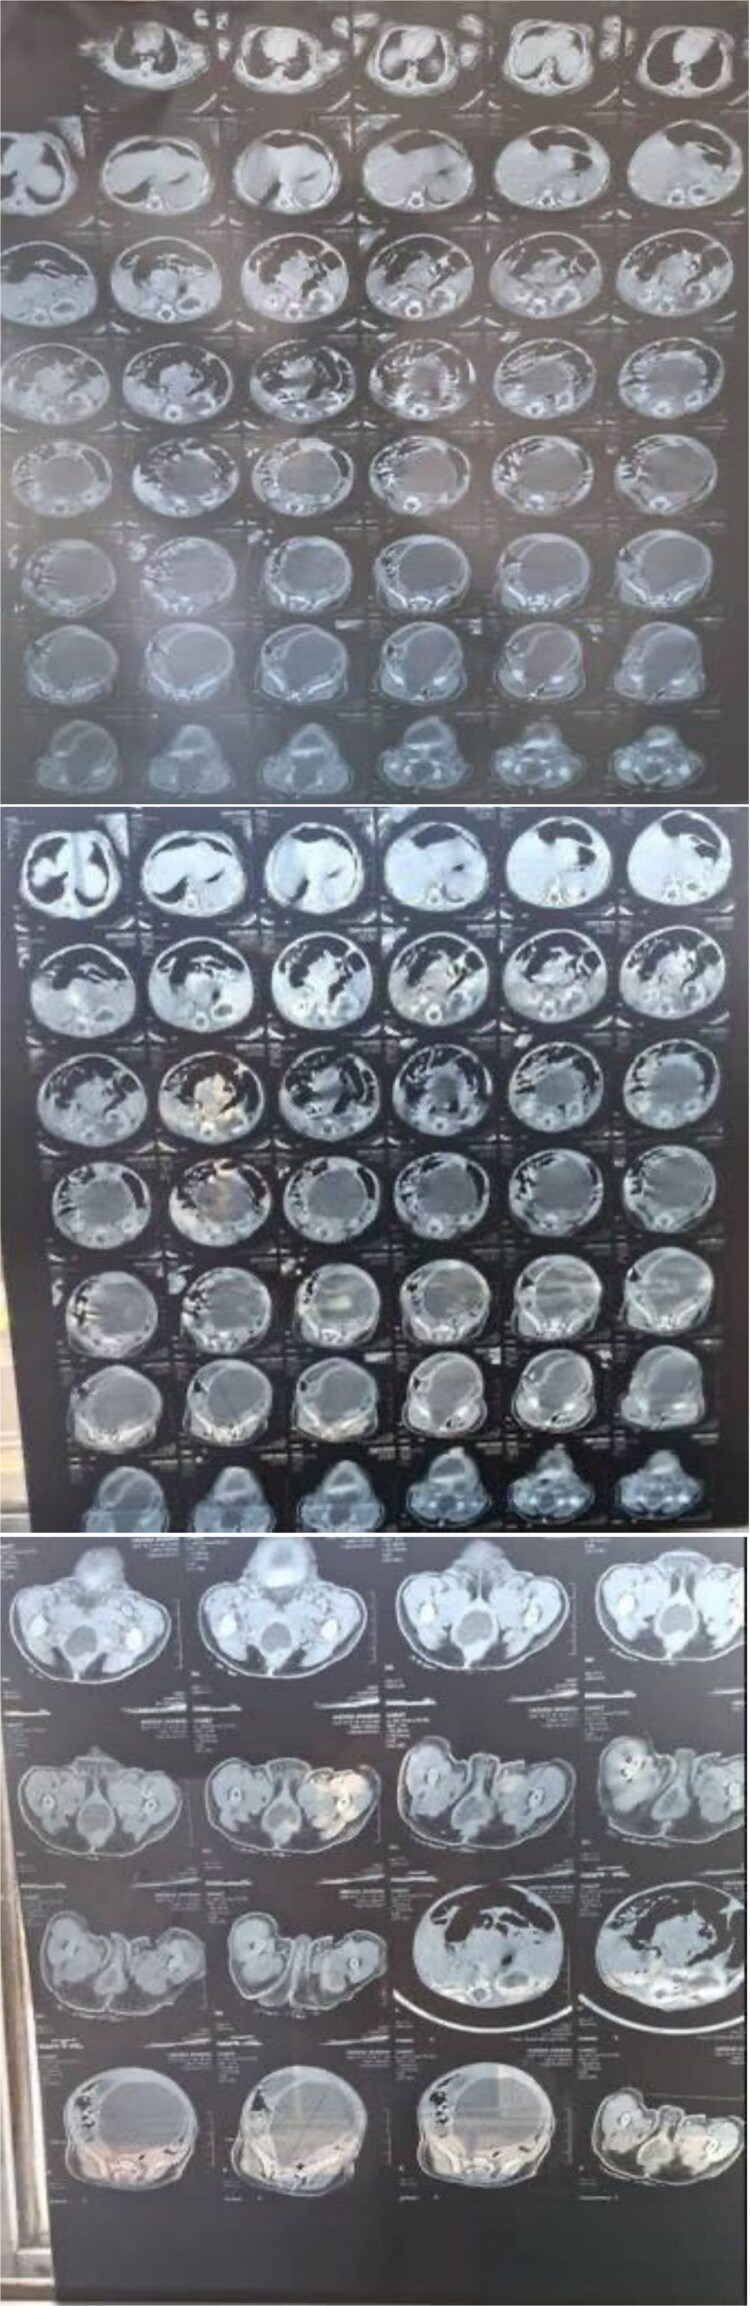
CT demonstrates a markedly distended, fluid-filled vaginal cavity in this infant, consistent with hydrocolpos, extending superiorly and exerting mass effect on adjacent pelvic organs, with secondary bilateral hydronephrosis.
